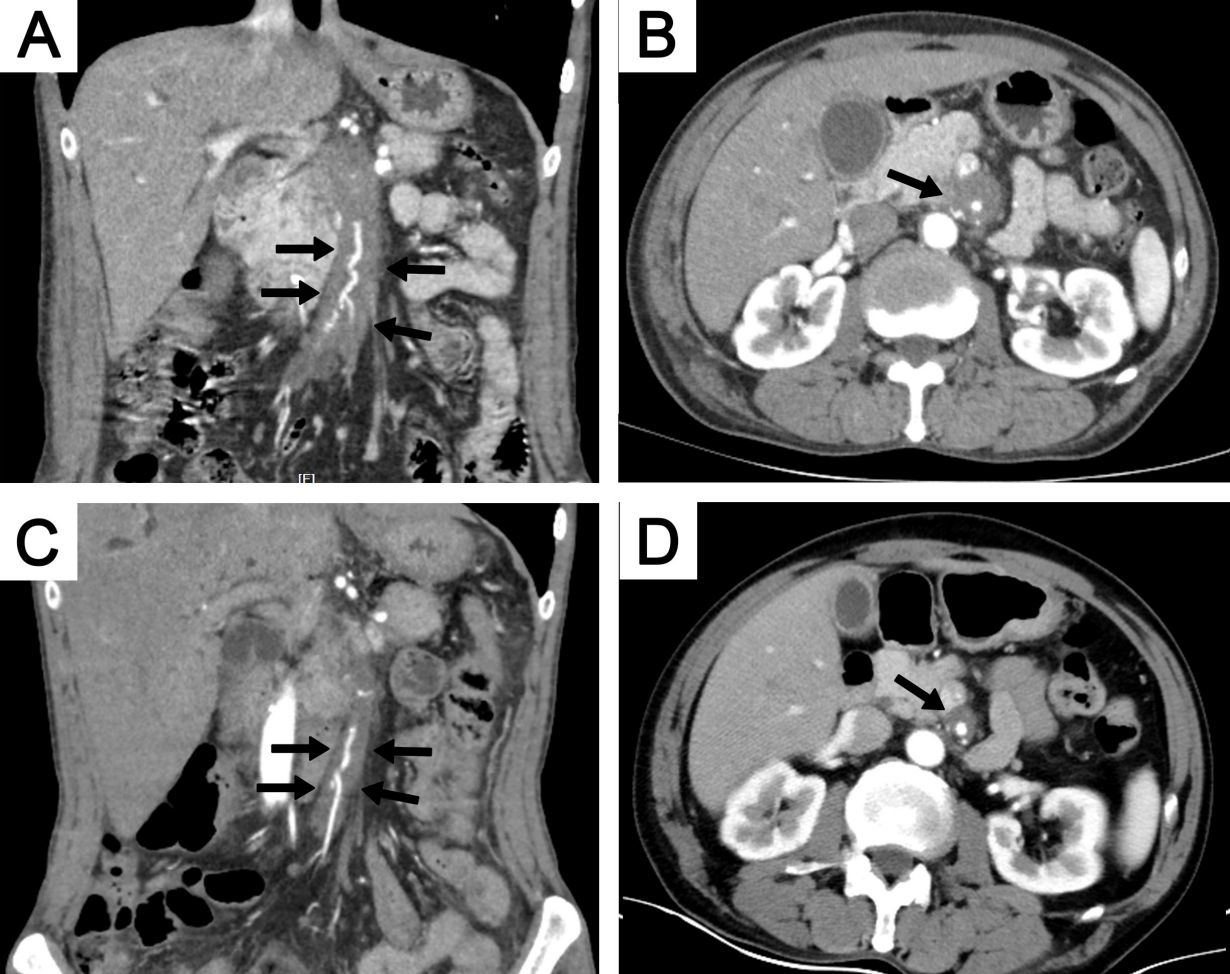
A 64-year-old man with involvement of the superior mesenteric artery. CECT showed soft tissue surrounding the superior mesenteric artery in both coronal (A) and axial (B) views (black arrows). The extent of the soft tissue decreased after 6 months of steroid treatment, as shown on coronal (C) and axial (D) views (black arrows). CECT, contrast-enhanced computed tomography.
